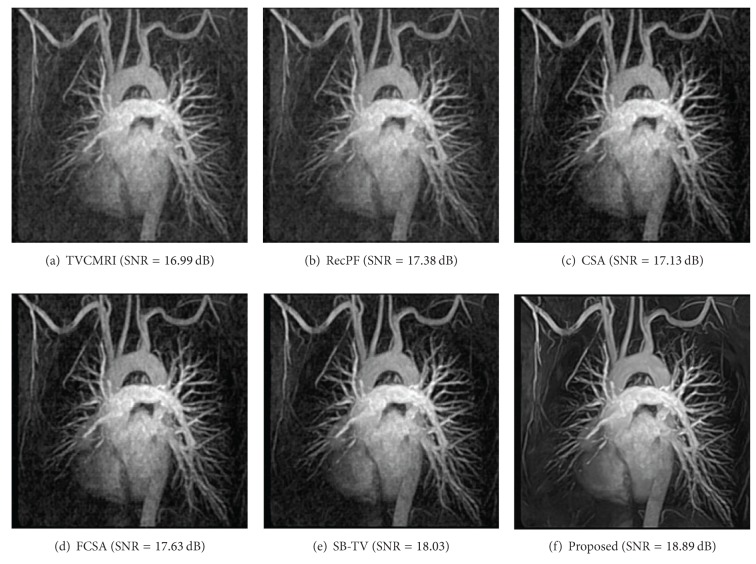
Chest MR image reconstruction from 20% samples (Gaussian noise case).
